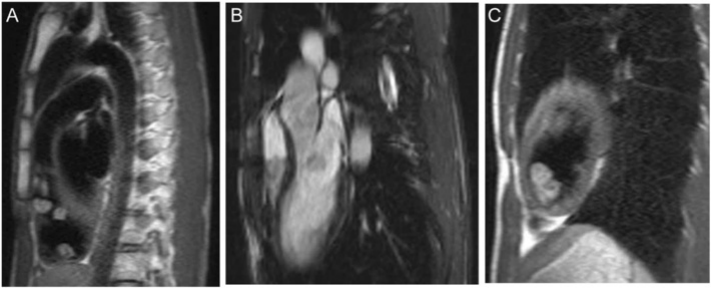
Identification of cardiac myxomas by MRI. The presence of cardiac myxomas was confirmed by MRI in patient 1. (A) Right ventricle. (B) Left atrium. (C) Left ventricle.
